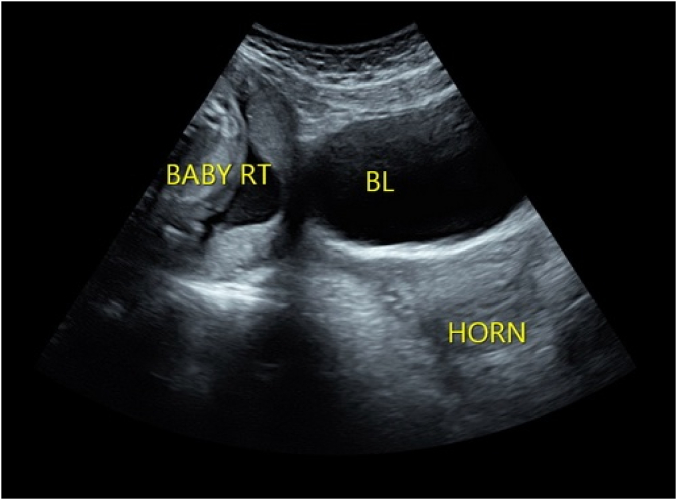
Ultrasound image from the dates and anatomy scan at 23 3/7 weeks of gestation with sonographer annotations. Baby RT demonstrates the pregnancy in the uterine horn on the maternal right, BL is the maternal bladder, and horn is the empty uterus.
